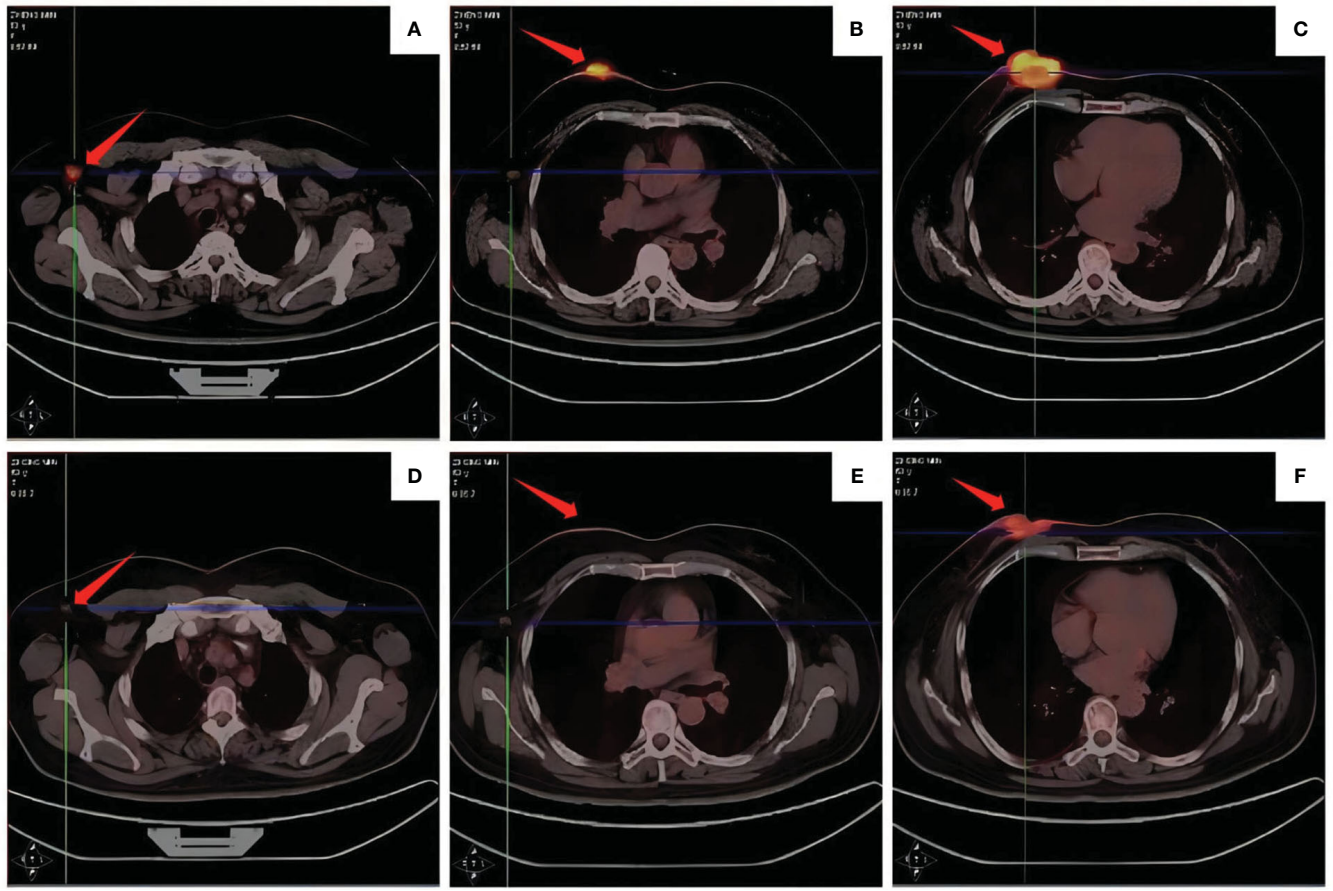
PET-CT results of patients at different time. On August 27, 2020, (A, B) multiple lymph nodes in the right axilla were enlarged, with a large diameter of about 1.5 cm, SUVmax: 9.1; (C) 3.7 cm x 3.8 cm tumor was seen in the right breast nipple, SUVmax: 22.4; (D-F) after four cycles of treatment (November 20, 2020), the right breast tumor and axillary lymph nodes were smaller than before.
